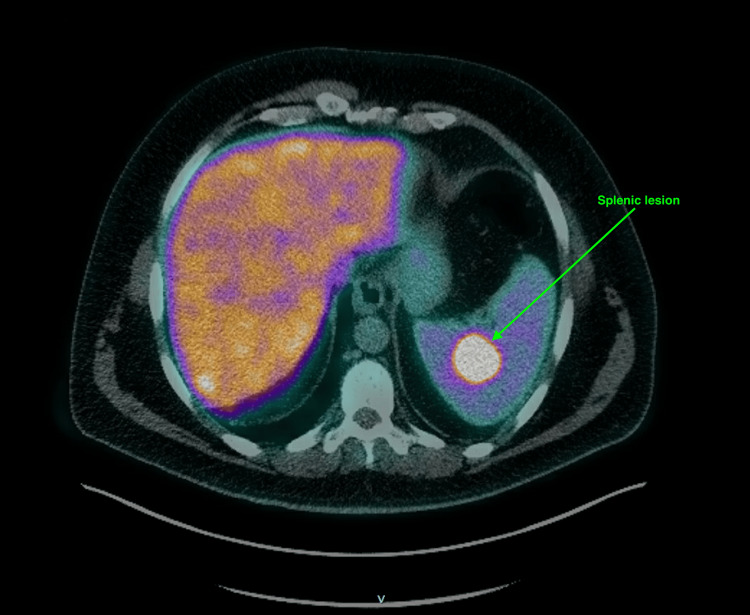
PSMA subtraction image showing radiotracer uptake in the spleen (green arrow). PSMA = prostate-specific membrane antigen
